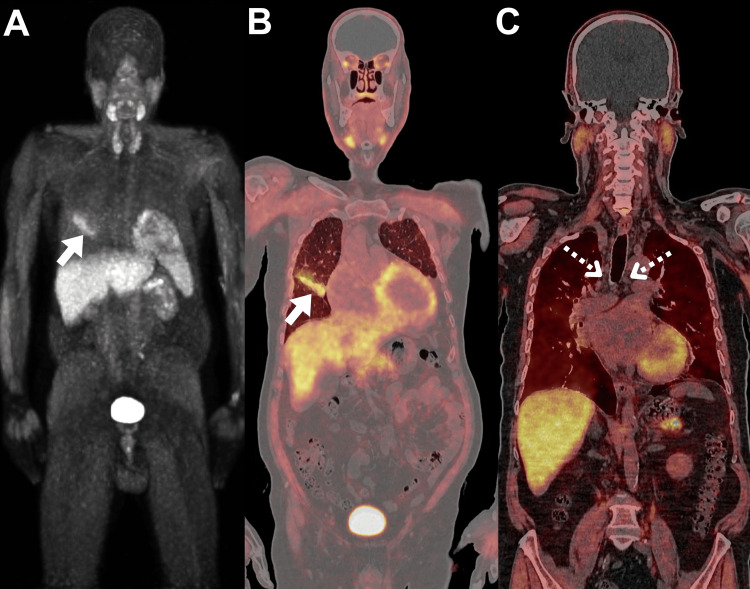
68Ga-FAPI PET/CT images of the patient. (A) MIP, and (B, C) coronal views of 68Ga-FAPI PET/CT images were obtained five days after initial 18F-FDG PET/CT scanning. The scan revealed the same right pulmonary mass with significant 68Ga-FAPI expression (SUVmax of 8.7; arrows). Notably, the previously observed bilateral mediastinal lymph nodes were unremarkable (SUVmax of 1.4, dotted arrows). MIP: maximal intensity projection; 68Ga-FAPl PET/CT: 68Ga-fibroblast activation protein inhibitor positron emission tomography/computed tomography; SUV_max_: maximum standardized uptake value.
